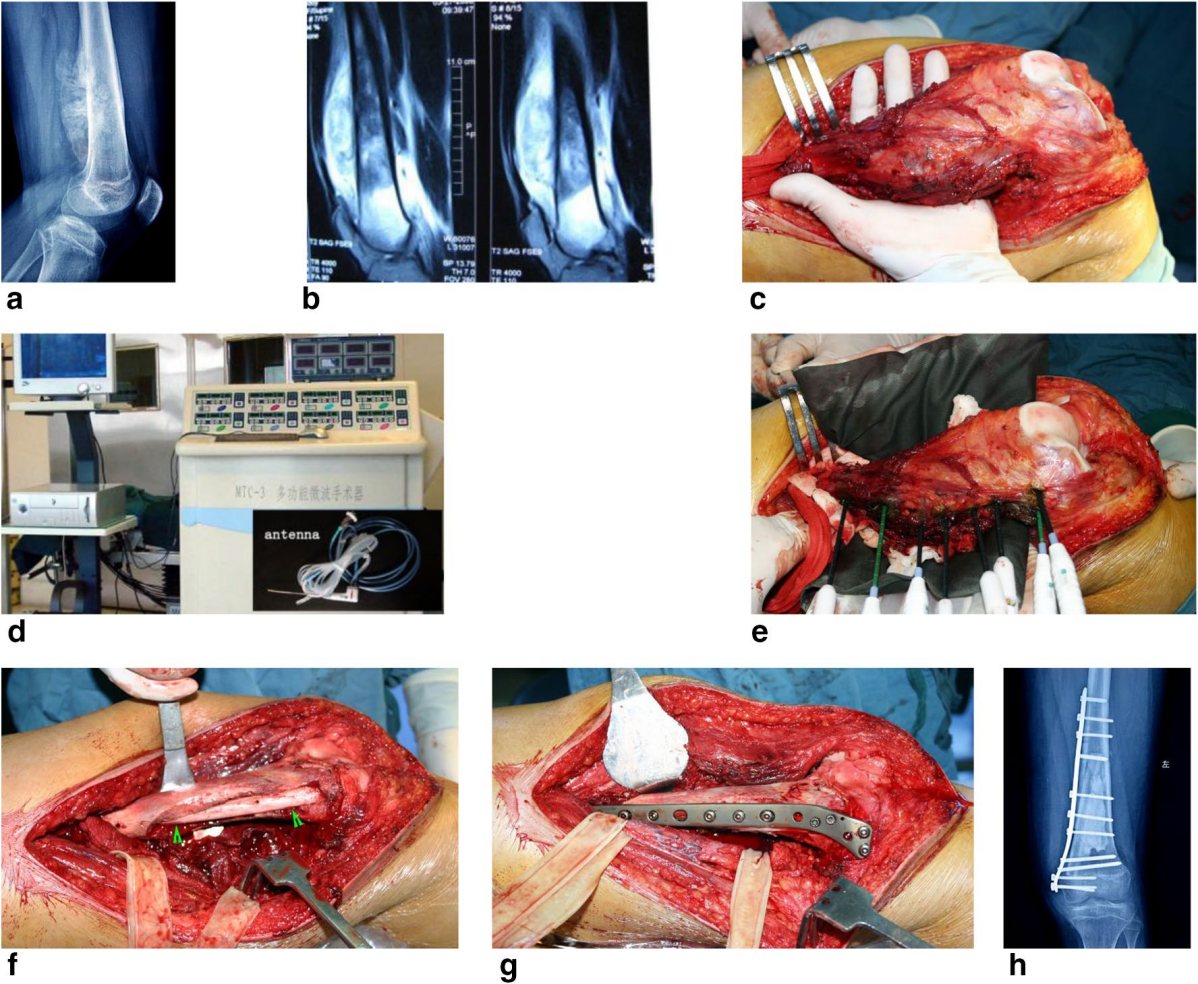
Typical procedure for osteosarcoma at distal femur. a, b Image data shows an osteosarcoma of distal femur. c Dissect the tumor-bearing bone from surrounding normal tissues with safe margin. d Put a heat-isolation pad between the tumor bone and surrounding normal tissues and began to deliver electromagnetic energy into tumor bone. e The microwave generator and antenna. f Remove or curettage the dead soften tumor mass and give fibular bone autograft. The mixture materials of bone chips and bone cement was used filling the cavity. g Restore the normal shape of the femur and give a prophylactic fixation. h X-ray film after surgery
